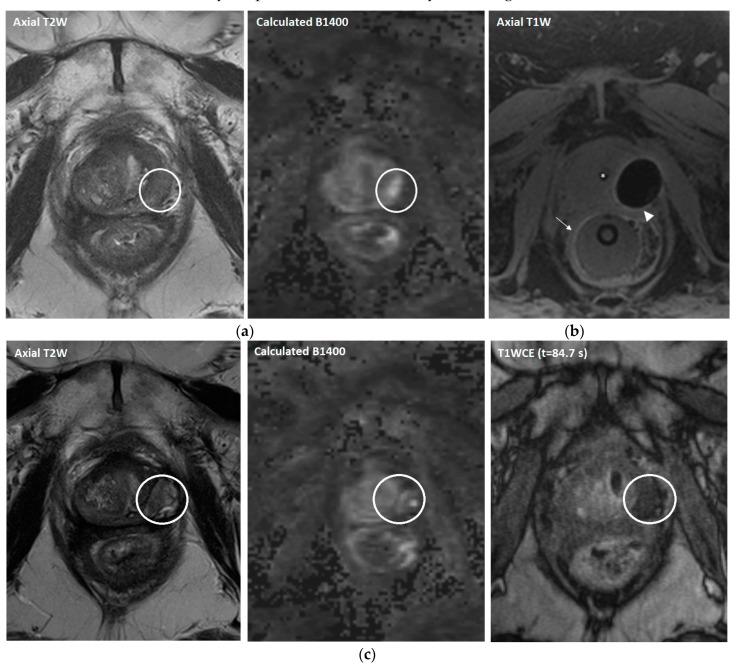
(a) Pre-treatment axial T2W and high-*b*-value MR images in a 61-year-old patient with recurrent prostate cancer in the peripheral zone of the left mid gland (circle) with a PSA level of 2.0 ng/mL (Gleason score 4 + 4 = 8) after initial treatment with external beam radiation therapy. (b) The axial T1W MR image demonstrates the cryoablation under near real-time guidance with two cryoneedles (ice-seeds). The urethra (*) and rectum (arrow) contain a urethral warming catheter and a rectal balloon, respectively. The iceball is sharply visualized as a signal void area surrounded by a hyperintense rim corresponding to 0 °C (arrowhead). (c) Post-treatment axial T2W, high-*b*-value, and contrast-enhanced MR images at 3 months follow-up after cryoablation (PSA level of 1.2 ng/mL) showing no evidence of residual disease or recurrence (circle).
